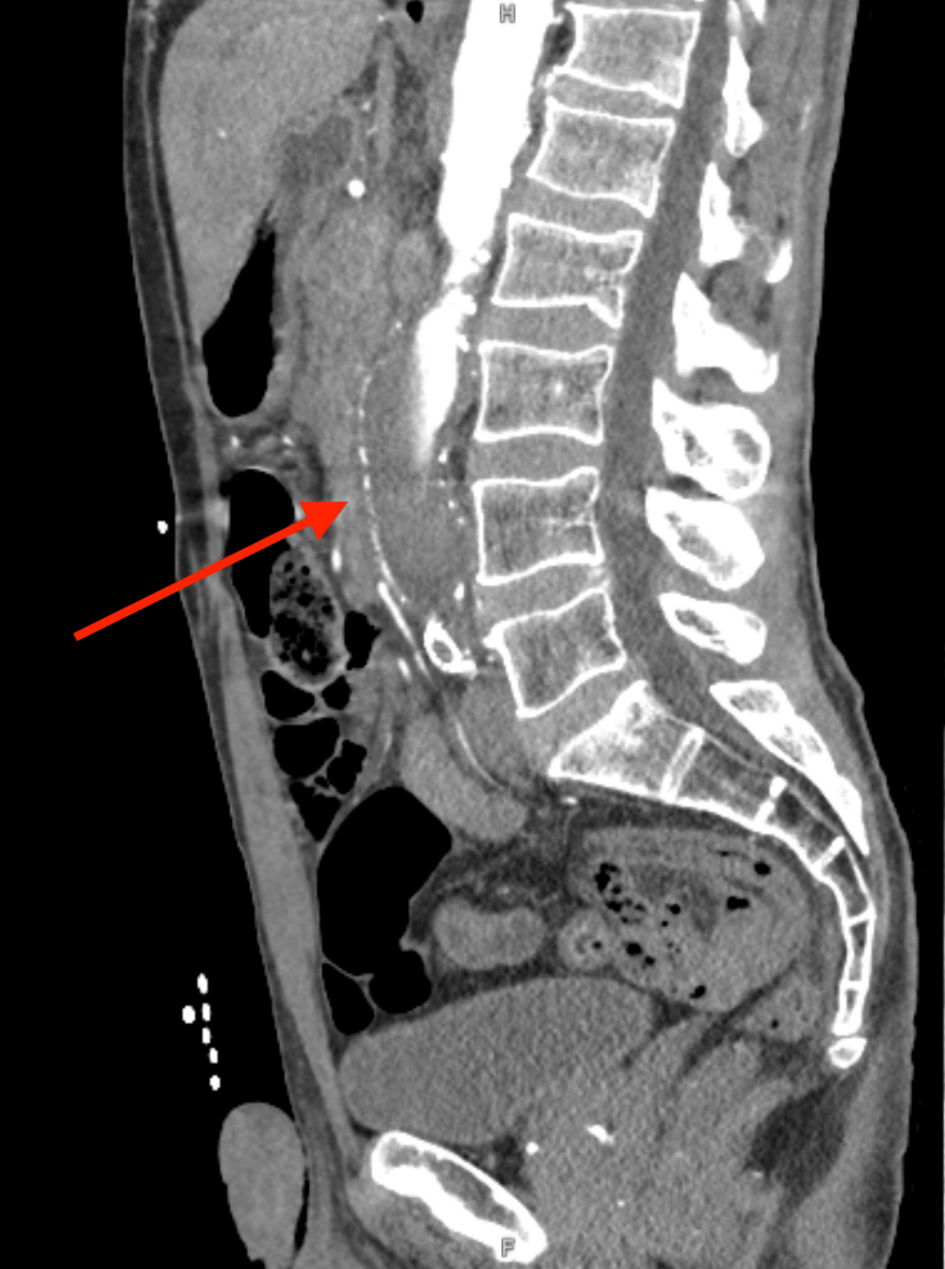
Sagittal image from CTA demonstrating the aneurysm (red arrow) CTA: computed tomography angiogram
